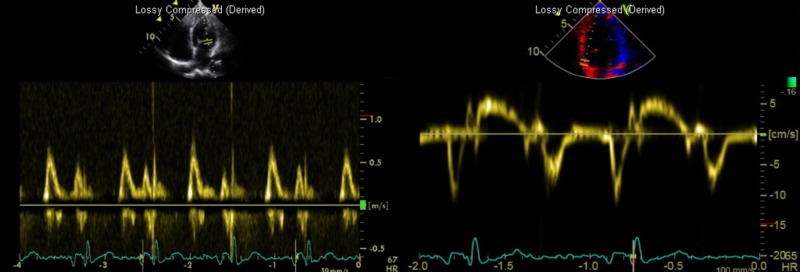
Echocardiogram imaging displaying mitral inflow Doppler (left) showing a normal E/A ratio and deceleration time. Tissue Doppler (right) shows an elevated e/e’ ratio indicative of Grade II (pseudonormal) diastolic dysfunction.
